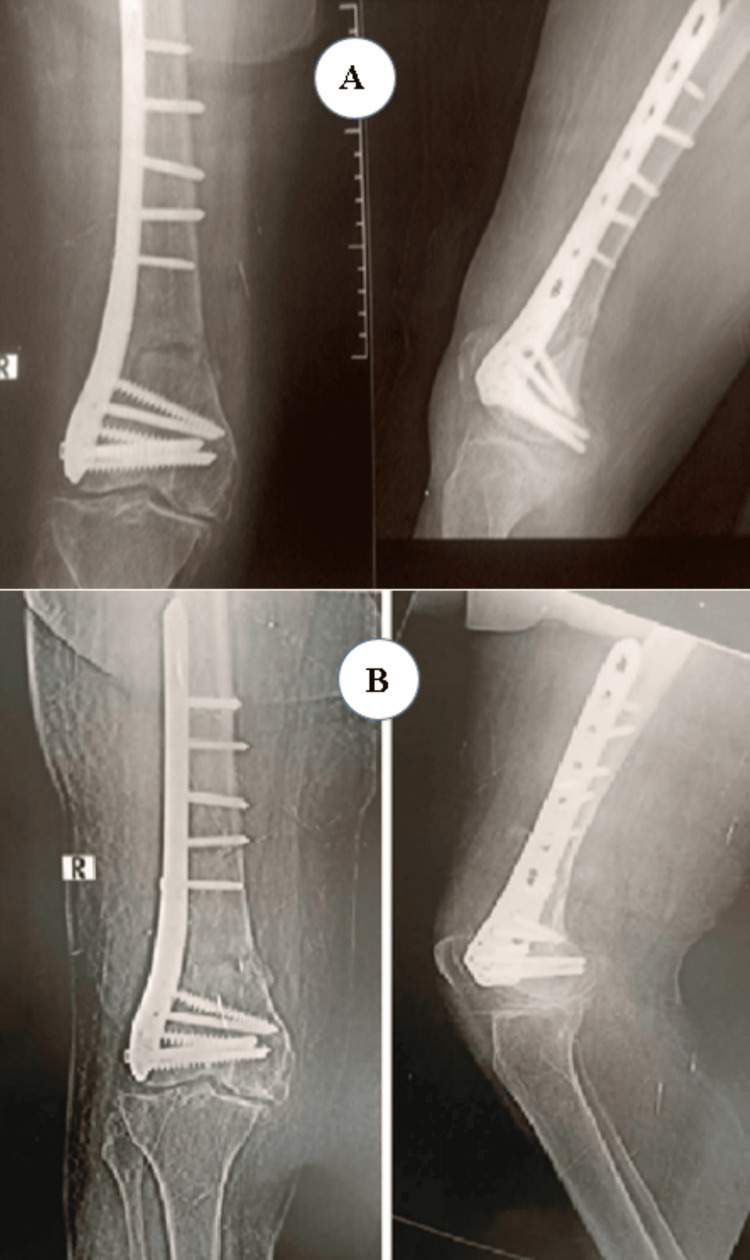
Case 3 follow-up X-rays at (A) three and (B) six months
